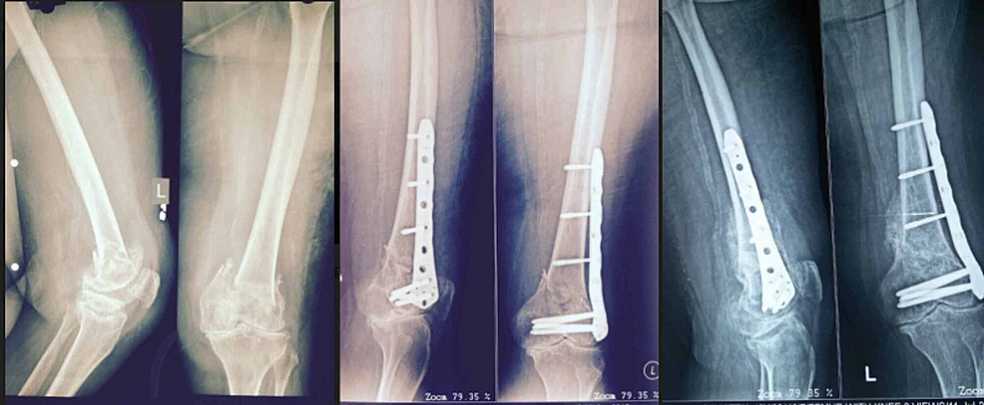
Preoperative, two weeks and six-month follow-up radiographs.
